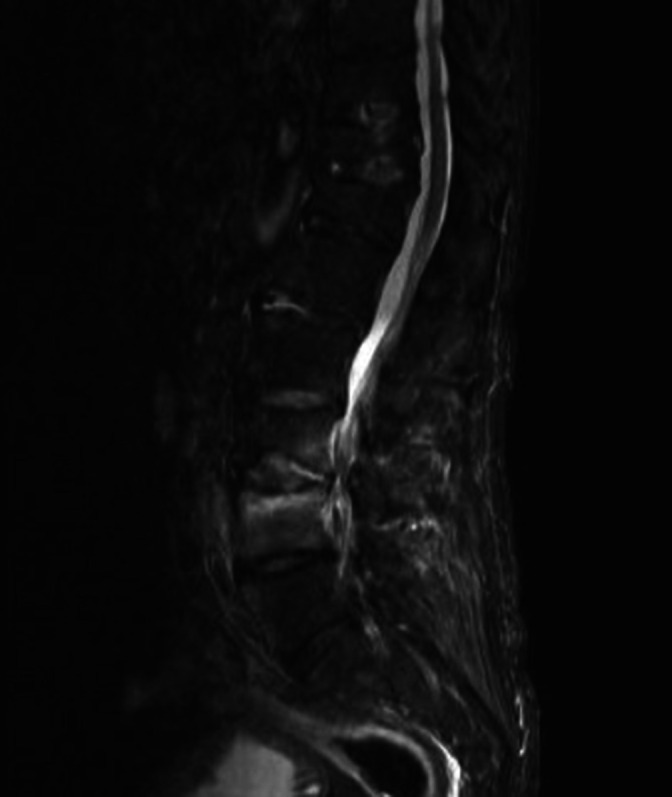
Sagittal MRI STIR sequence of the lumbosacral spine showing hyperintensity in L4 vertebral body and L3/4 disc space.
